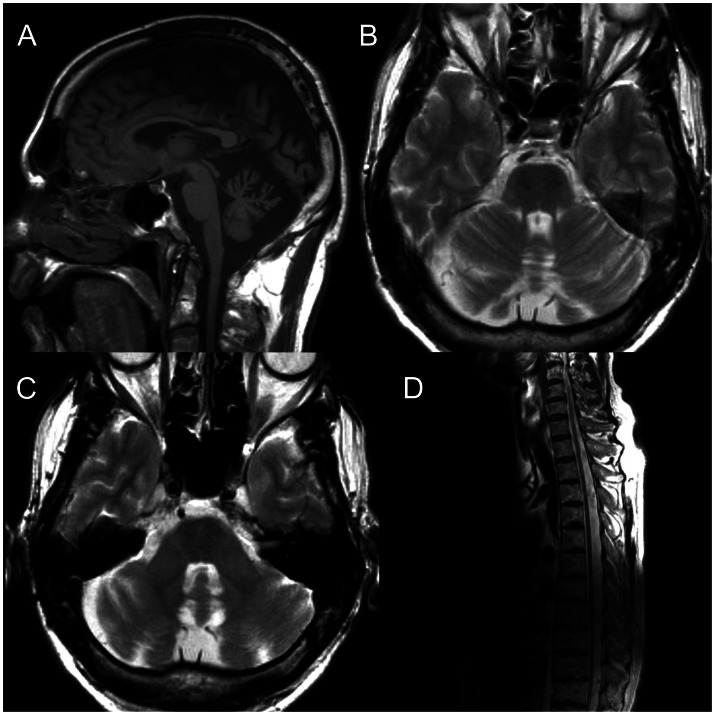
MRI of the index patient at the age of 54 years. A. Sagital T1 weighted MRI of the brain showing atrophy of the cerebellar midline, particularly the vermis superior. B&C. Axial T2 weighted MRI showing linear T2 hypointensities in the pons. C. Axial T2 weighted MRI showing prolongation of T2 signal in the dentate nuclei. D. Sagital T2 weighted MRI showing atrophy of the cord, straight dorsal spine and loss of the dorsal kyphosis.
